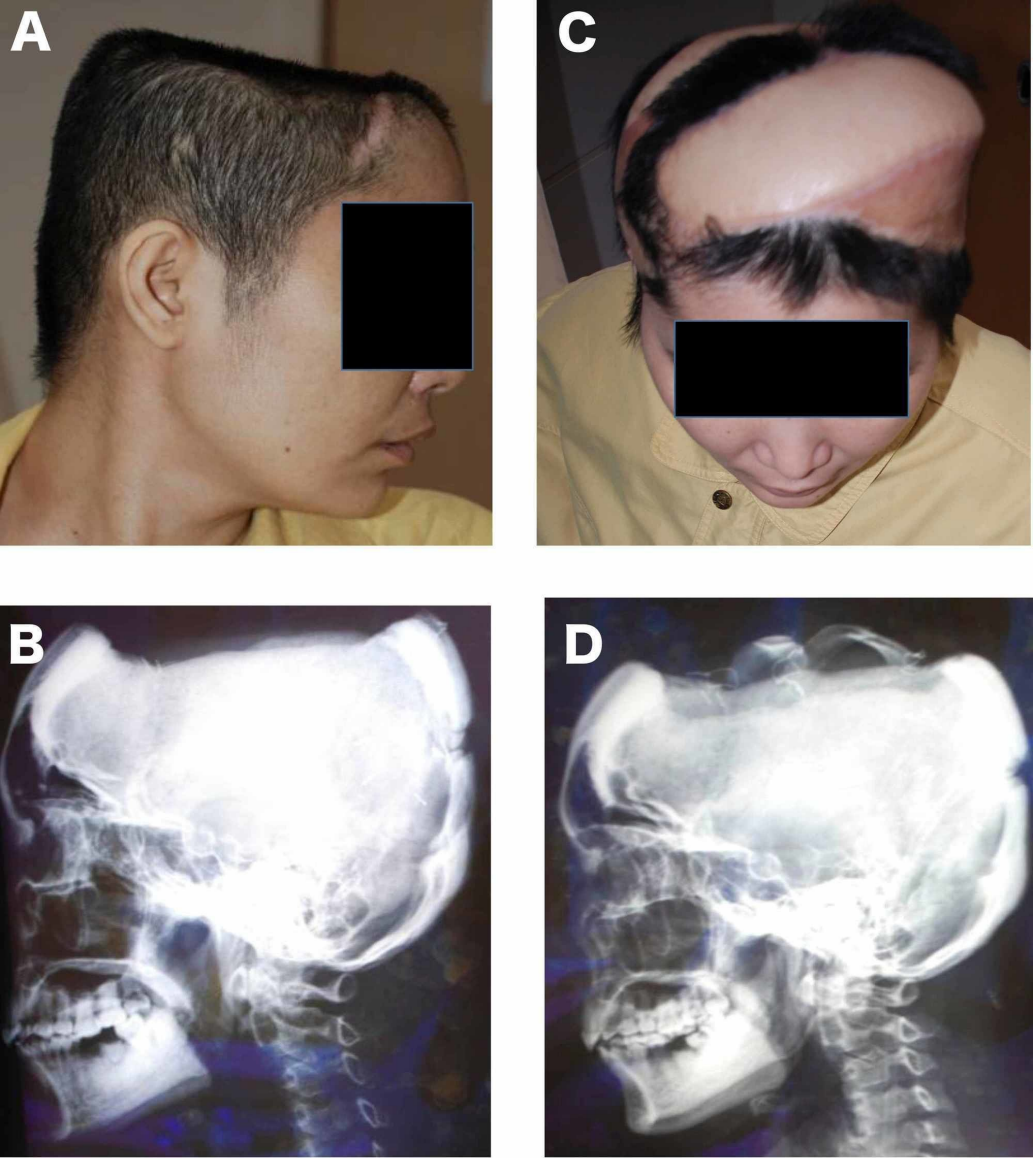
Scalp and calvarium reconstruction with extended fleur-de-lis LD musculocutaneous free flap (A) Preoperative view of the patient; (B) Preoperative X-ray; (C) Postoperative view; (D) Postoperative X-ray showing the calvarium reconstruction with two ribs.
